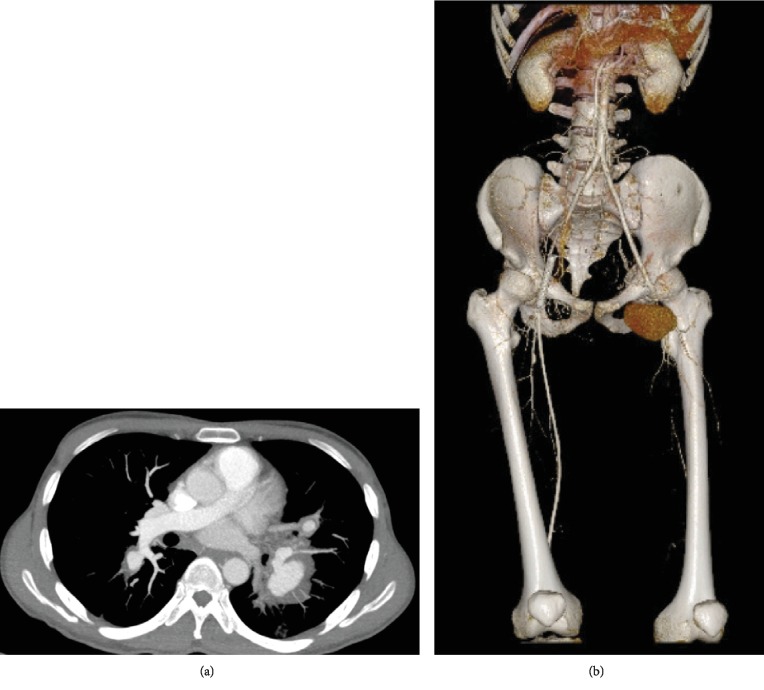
(a) Contrast-enhanced computed tomography of pulmonary artery showed a 33-year-old male BD patient with multiple pseudoaneurysms in bilateral pulmonary arteries. (b) Computed tomography with angiography of lower extremities showed a 19-year-old male BD patient with pseudoaneurysm on the left superficial femoral artery.
